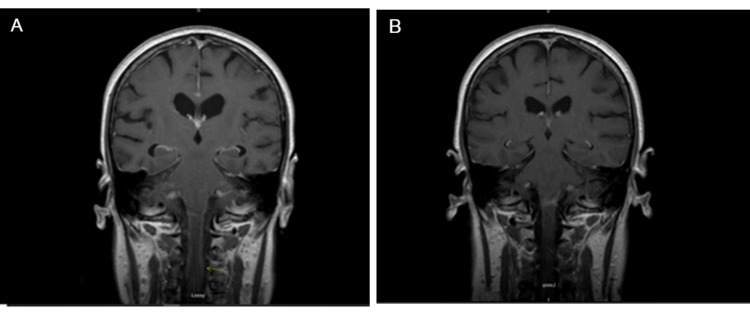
MRI brain and cervical spine showing leptomeningeal enhancement (yellow arrow) at the time of presentation (A) and no leptomeningeal enhancement 2 years after systemic therapy (B)
